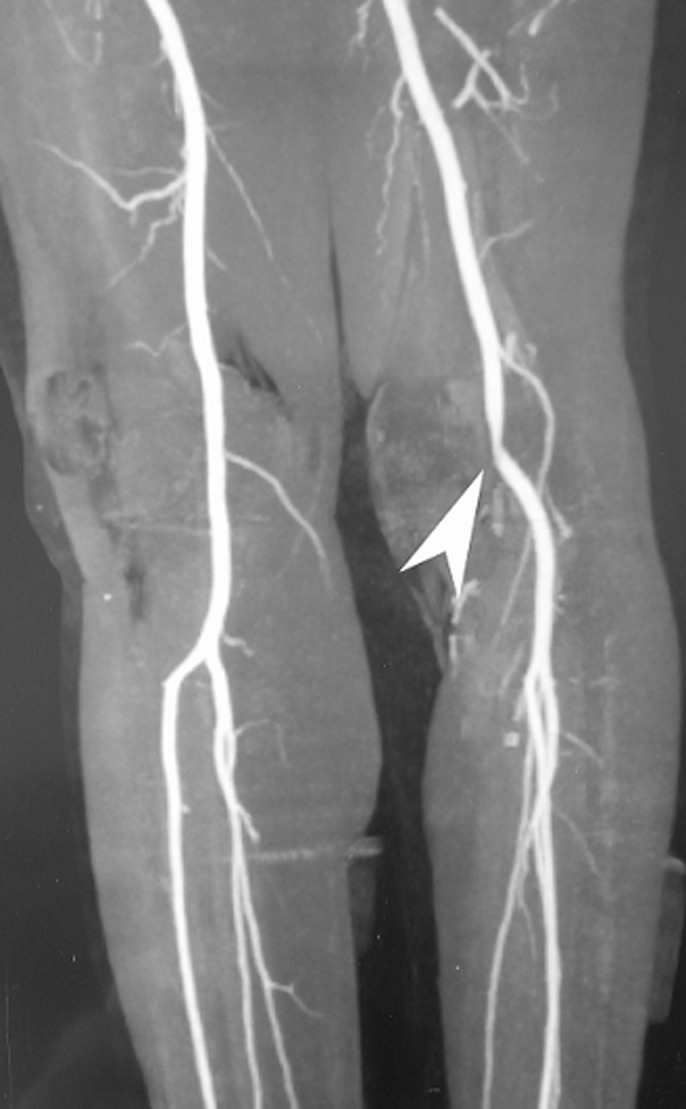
CT Angiography showing a partial occlusion of the popliteal artery due to the entrapment syndrome (PAES)
